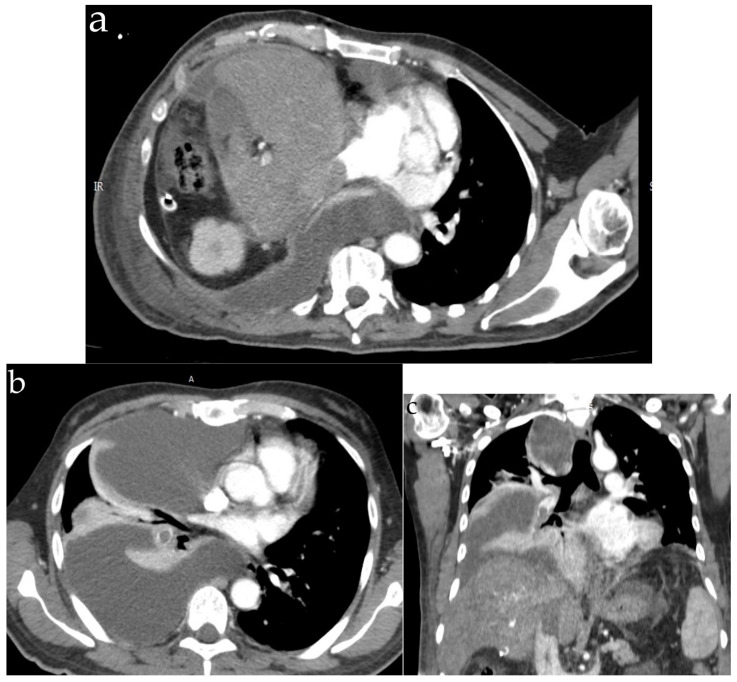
Thoraco-abdominal CT: hepatocellular carcinoma—local recurrence with enhancing tumoral thrombus into the inferior vena cava (a) and tumoral emboli introduction into the inferior right lobe branch of the pulmonary artery (b,c).
